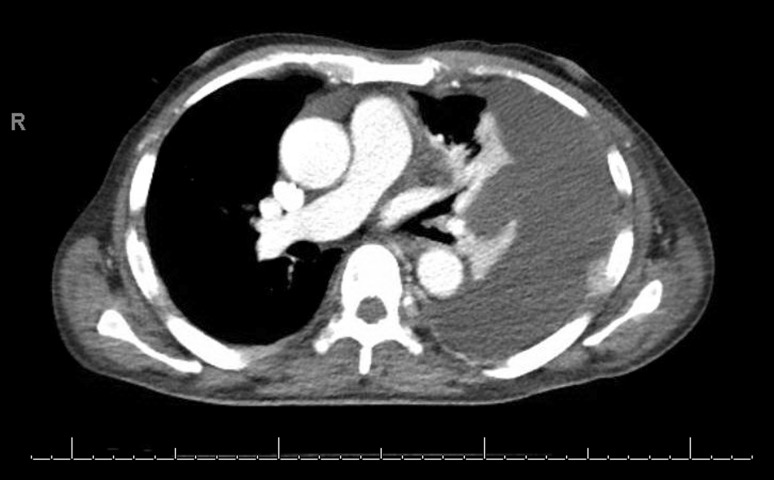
Chest computed tomography (CT) with intravenous contrast demonstrating malignant left pleural effusion with left lower lobe collapse and segmental atelectasis in left upper lobe
